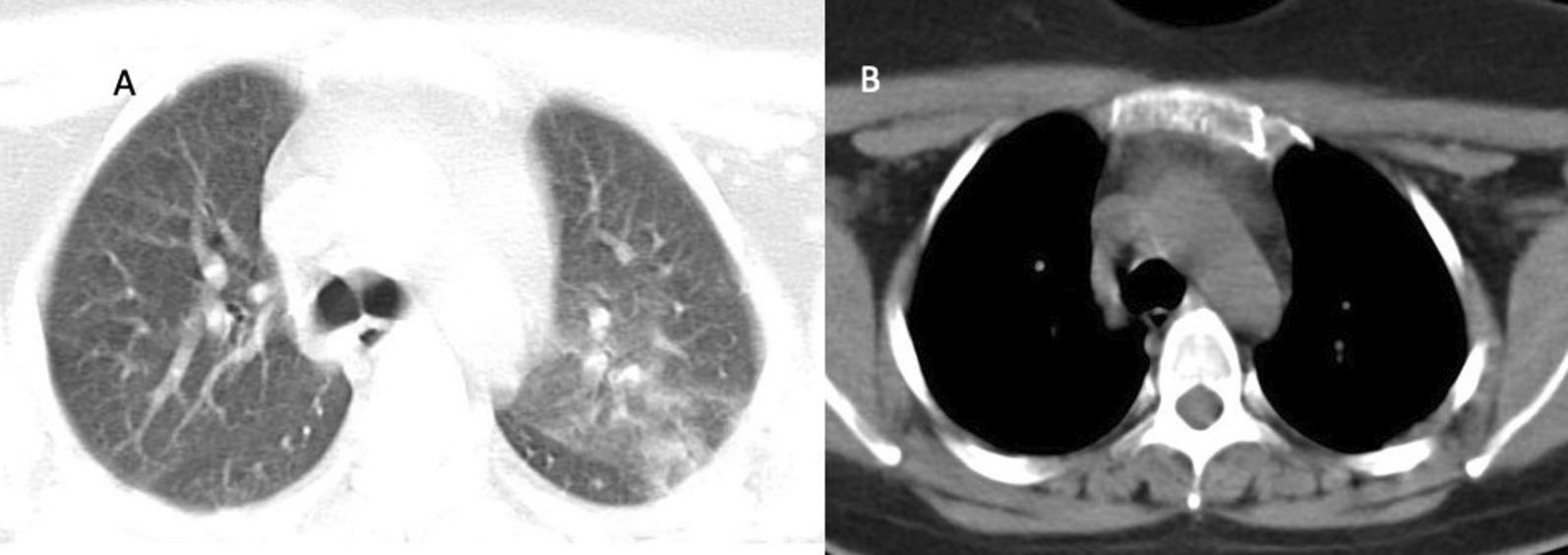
29-year-old female COVID-19(+) patient with CT-SS 12 (A) and nodular-type thymus (B)
